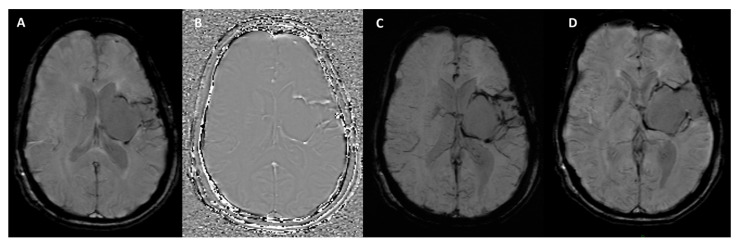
SWI sequence outputs from the Siemens MAGNETOM Aera (Erlangen, Germany) 1.5T MRI scanner in a patient with hemosiderin deposits surrounding a surgical cavity in the left cerebral hemisphere. (A) Magnitude Image: displays areas of hypointense signal indicative of hemosiderin deposits. (B) Phase Image: shows the hemosiderin deposits as hyperintense structures, highlighting their magnetic susceptibility effects. (C) SWI-Processed Image: combines magnitude and phase data to enhance visualization of susceptibility artifacts, such as the hemosiderin rim around the surgical cavity. (D) Minimum Intensity Projection: highlights the distribution of low-signal susceptibility-related structures, improving the visualization of hemosiderin deposits and venous anatomy.
